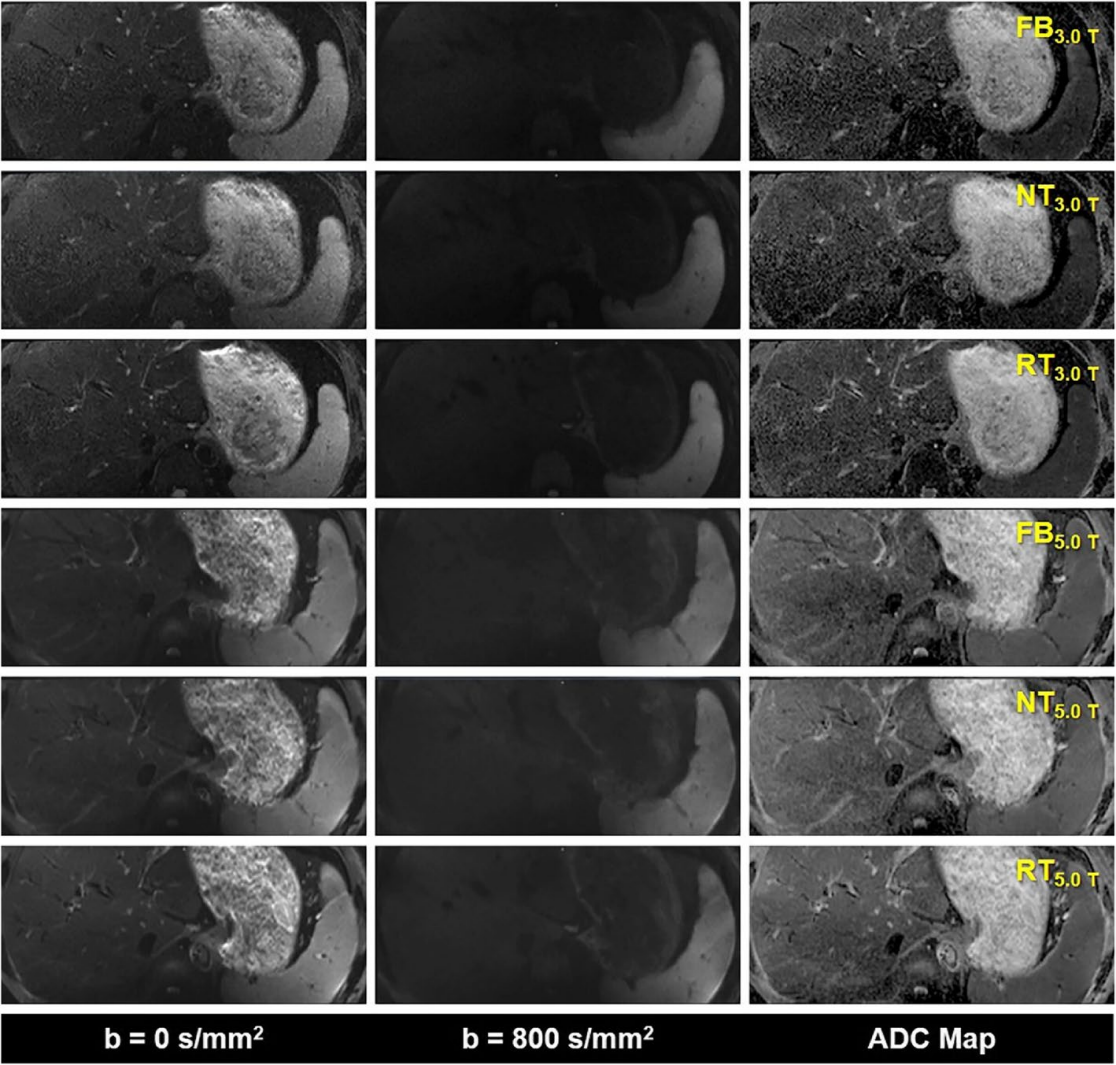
Representative MR images of an 18-year-old man weighing 74.2 kg and with a BMI of 25.4. The DWI images obtained by six acquisition approaches are listed in different rows. The DWI images of *b* = 0 s/mm^2^, *b* = 800 s/mm^2^, and ADC parametric maps are displayed in different columns
